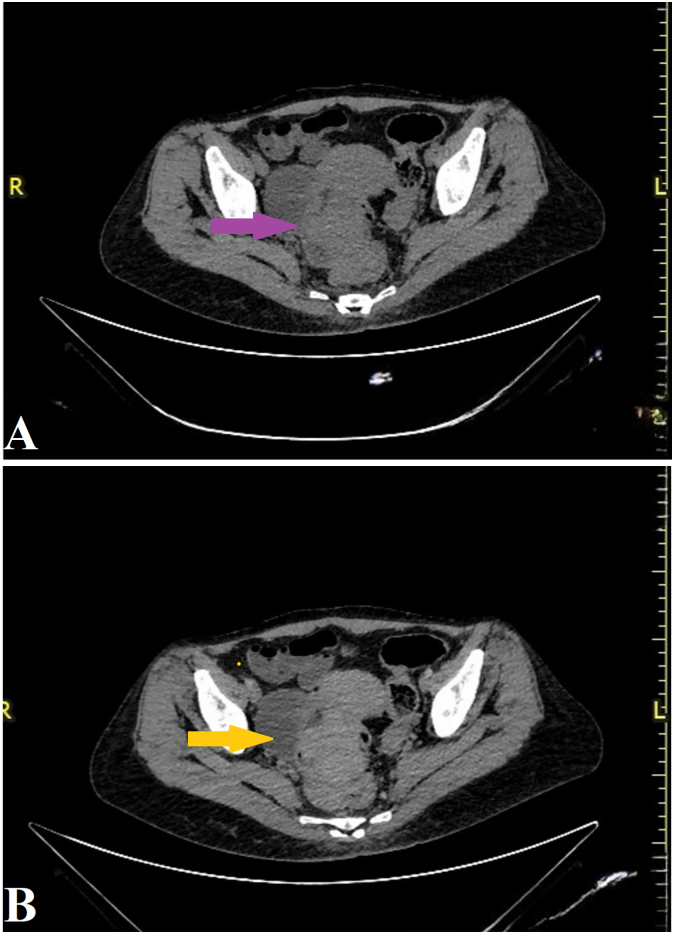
Abdominal computed tomography (CT) scan. (A) Axial non-contrast CT image showing a large, irregular polypoid mass (purple arrow) at the rectosigmoid junction. (B) Axial contrast-enhanced CT image demonstrating heterogeneous enhancement of the mass (golden arrow), measuring approximately 60 × 53 × 52 mm. (For interpretation of the references to colour in this figure legend, the reader is referred to the web version of this article.)
